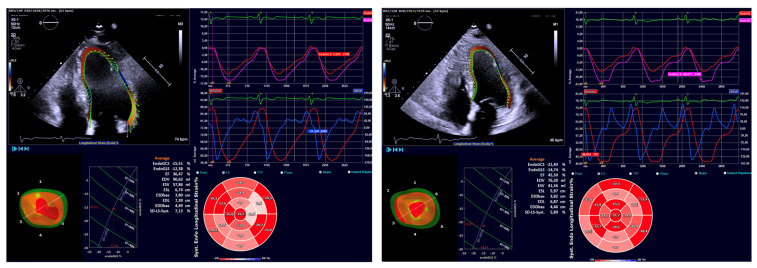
Changes in myocardial deformation parameters of LV assessed by STE after 3 months of iSGLT2 therapy. Baseline (t0) on the left; 3 months (t1) on the right. At each time point: (1) Top left: endocardial tracking; (2) bottom left: GCS; (3) bottom right: GLS; (4) top right: evolution over time of endomyocardial deformation (top) and volumes (bottom). GCS: global circumferential strain; GLS: global longitudinal strain; LV: left ventricle; STE: speckle tracking echocardiography.
